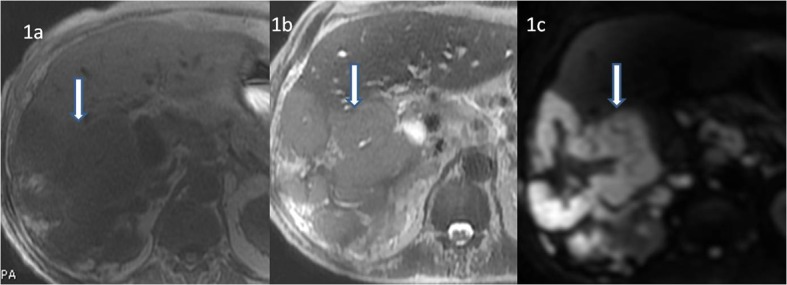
Non-contrast MRI sequences of the liver showing lesions in both lobes. (a) Lesions appear hypointense (white bold arrow) on T1-weighted sequence of non-contrast MRI. (b) Lesions appear hyperintense on T2-weighted sequence (bold white arrow). (c) Lesions show restriction (bold white arrow) on diffusion weighted images.
